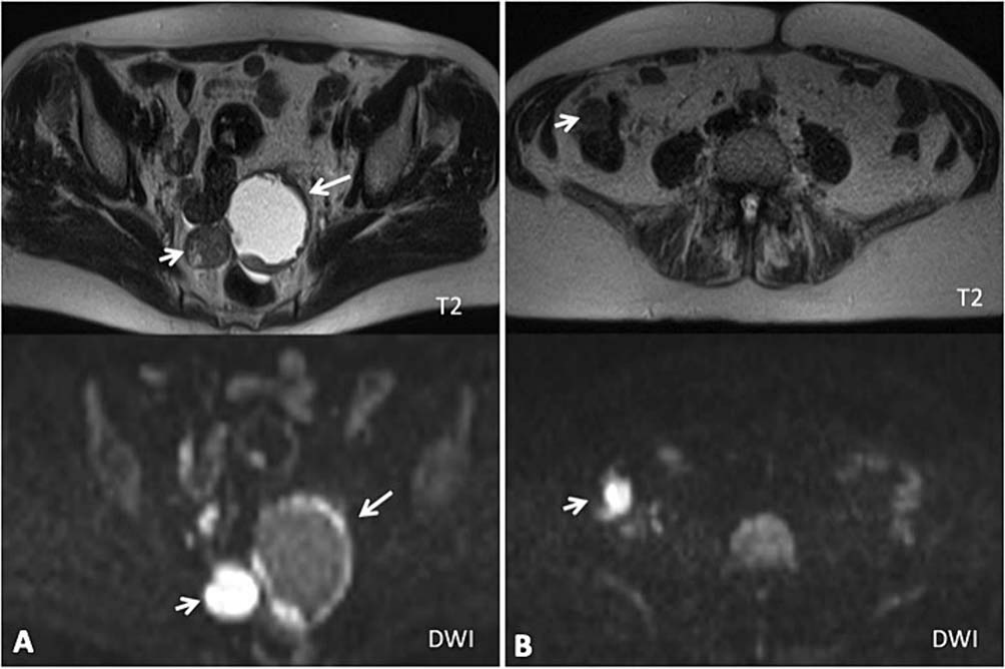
(A) Axial MRI imaging showing the pelvic tumor implants; (B) axial MRI imaging showing the implants in the right colon (diffusion weighted imaging).
